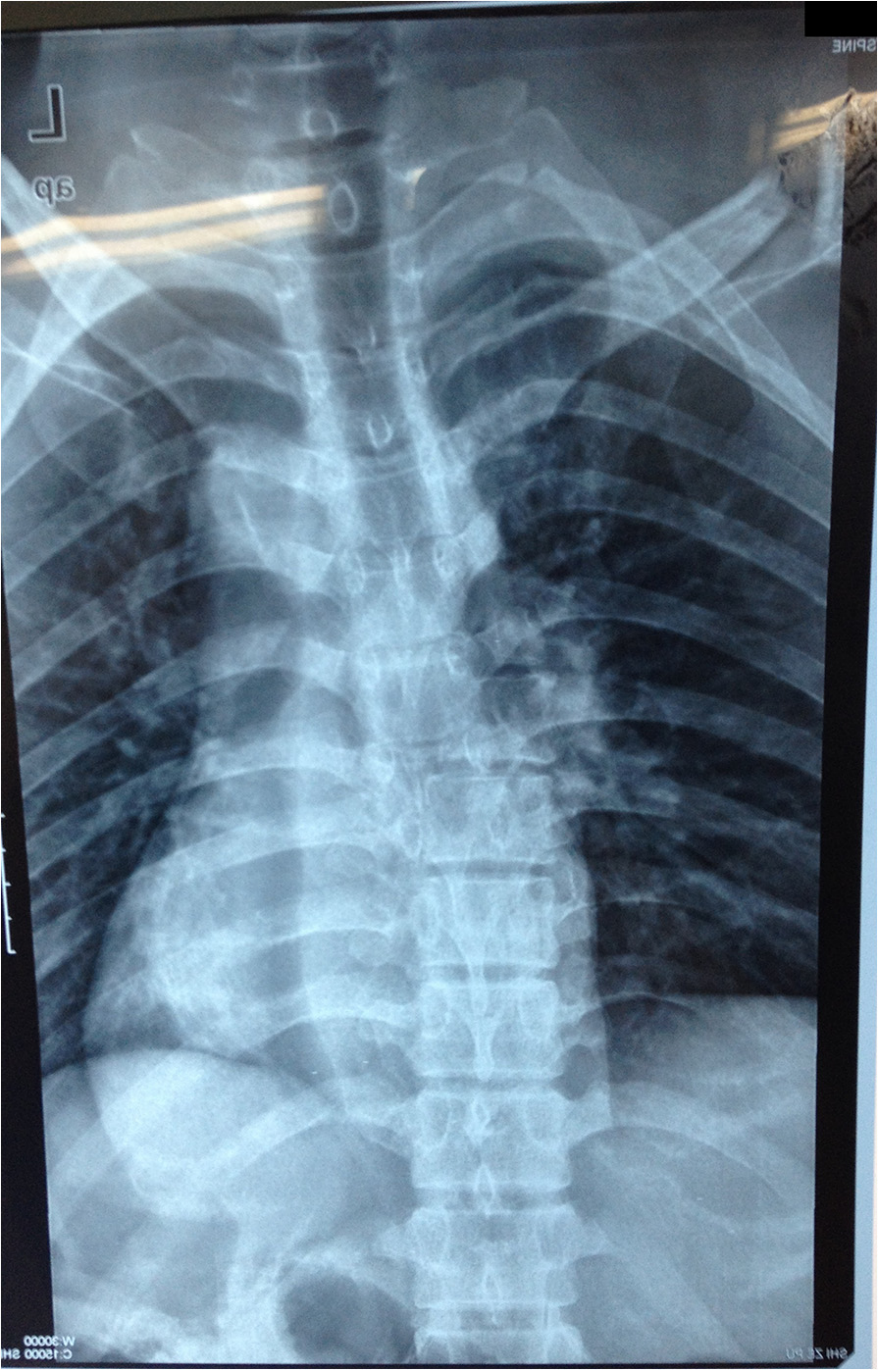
Anteroposterior thoracic spine plain X-ray showed spinal fracture-dislocation of T6 to T7.
